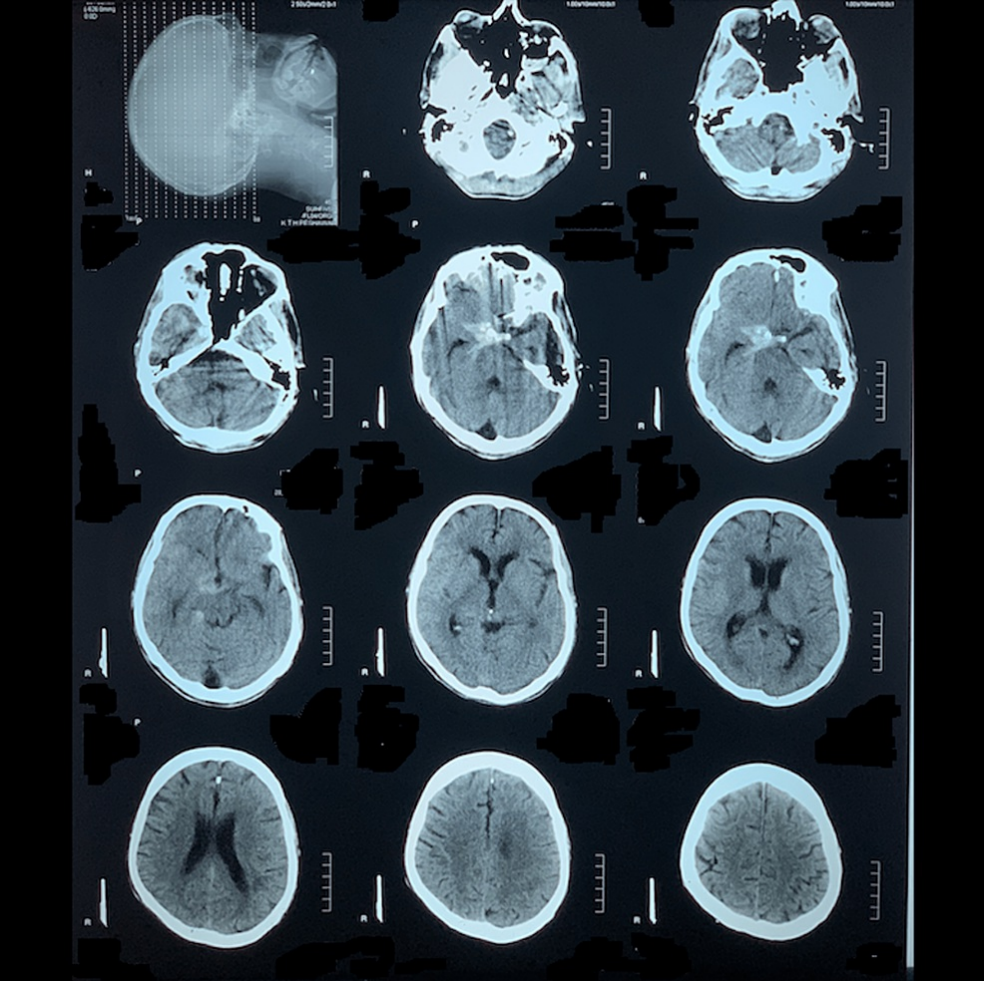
Computed tomography (CT) scan without contrast, findings of hyper-densities along the basal cisterns and right Sylvian fissure (more marked in suprasellar cistern).
